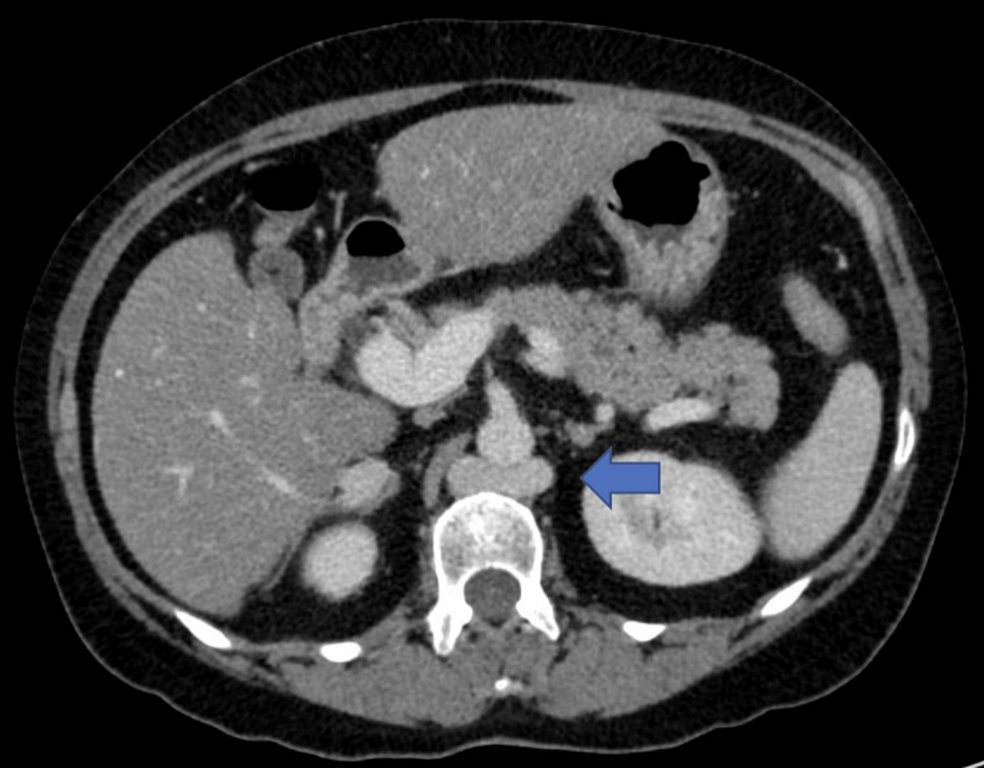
CT abdomen and pelvis, axial reconstruction, shows left renal vein draining into the dilated azygous system (blue arrow)
